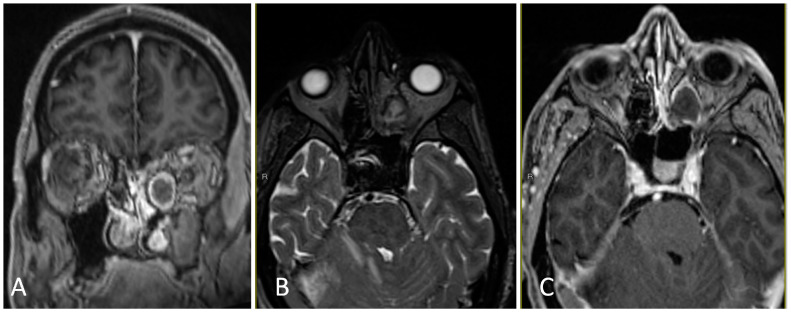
Postoperative imaging demonstrating complete resect of a left intraconal metastatic lesion and endoscopic endonasal optic nerve decompression. (A) Coronal T1 post-contrast MRI. (B) Axial T2-weighted MRI. (C) Axial T1 post-contrast MRI.
